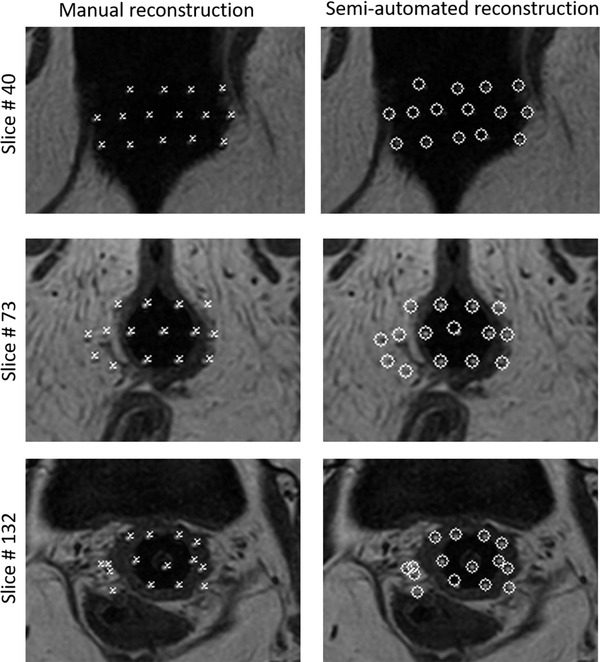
Axial T1‐Weighted MR image of a gynecological patient treated with 15 interstitial high dose rate (HDR) catheters. Left: manual reconstruction; (right) semiautomated reconstruction using the proposed algorithm
